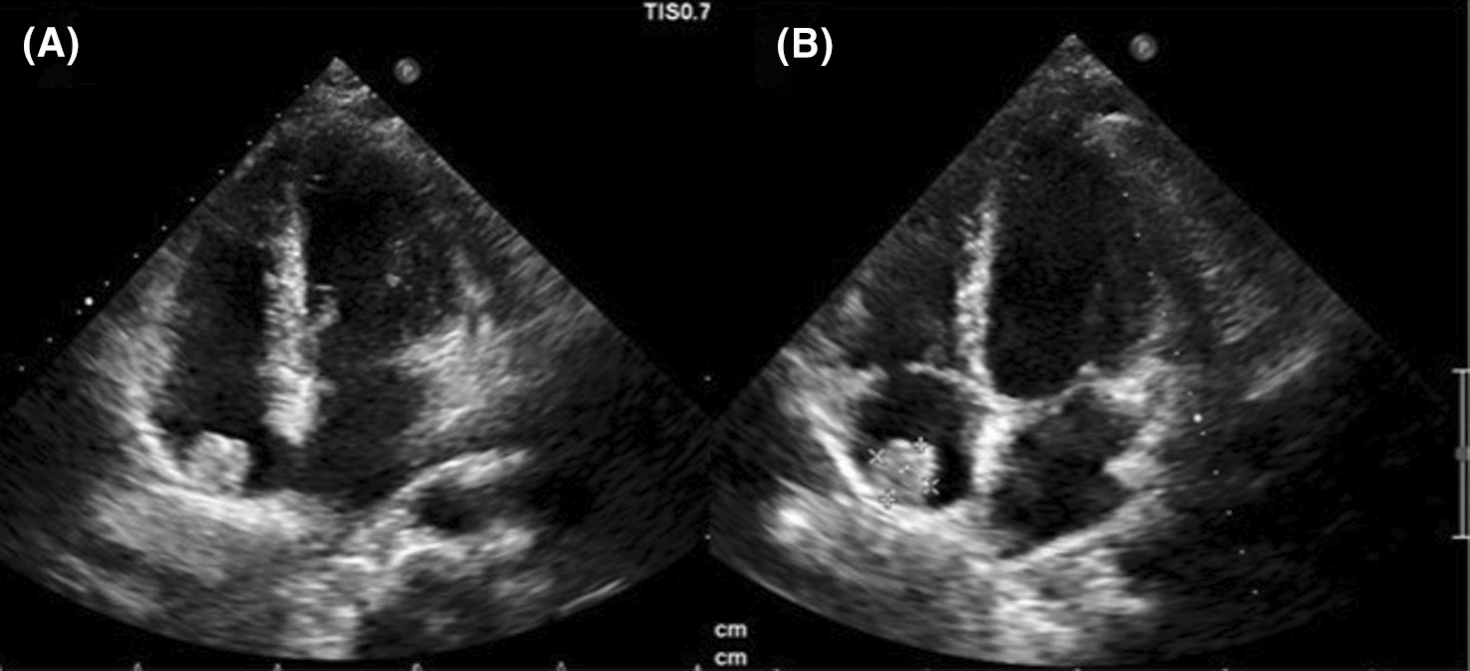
Follow‐up transthoracic echocardiography.
